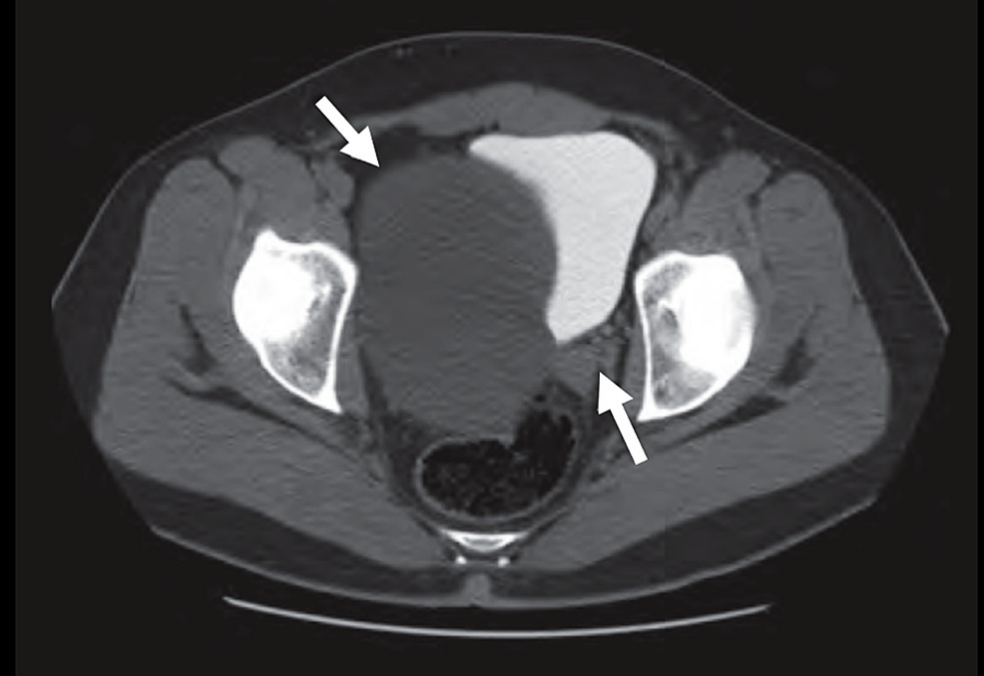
Axial computed tomography image. Axial contrast-enhanced computed tomography image of the pelvis (excretory phase) showing a well-defined cyst in the pelvis displacing the urinary bladder to the left (arrows).
